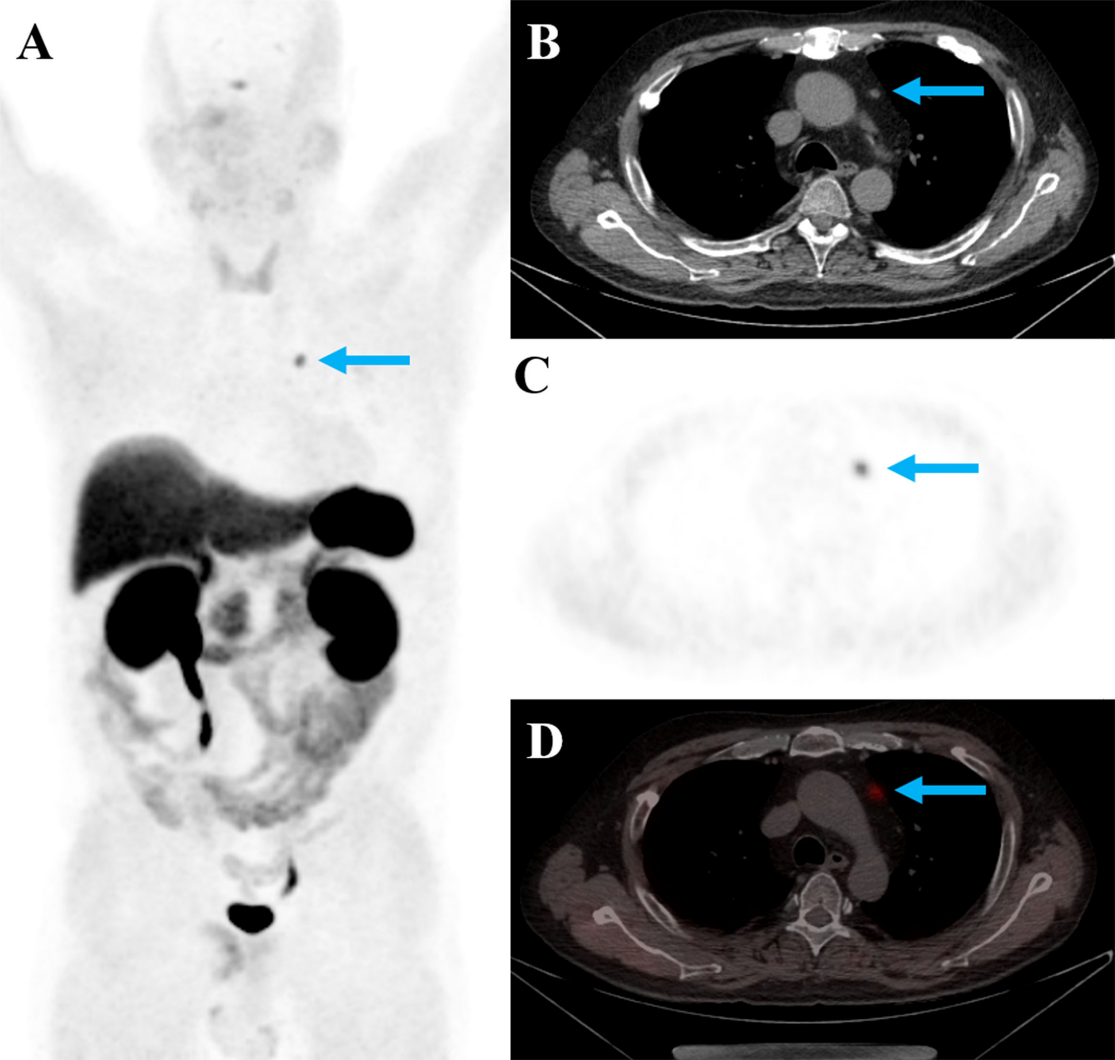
A 53-year-old man with EAS showed positive ^68^Ga-DOTATATE uptake in the thymic nodule (A–D, blue arrows; tumor SUVmax: 5.2; tumor size: 0.5cm). The nodule was surgically resected, and was pathologically confirmed to be thymic neuroendocrine tumor (G1, Ki-67 index, 1%).
